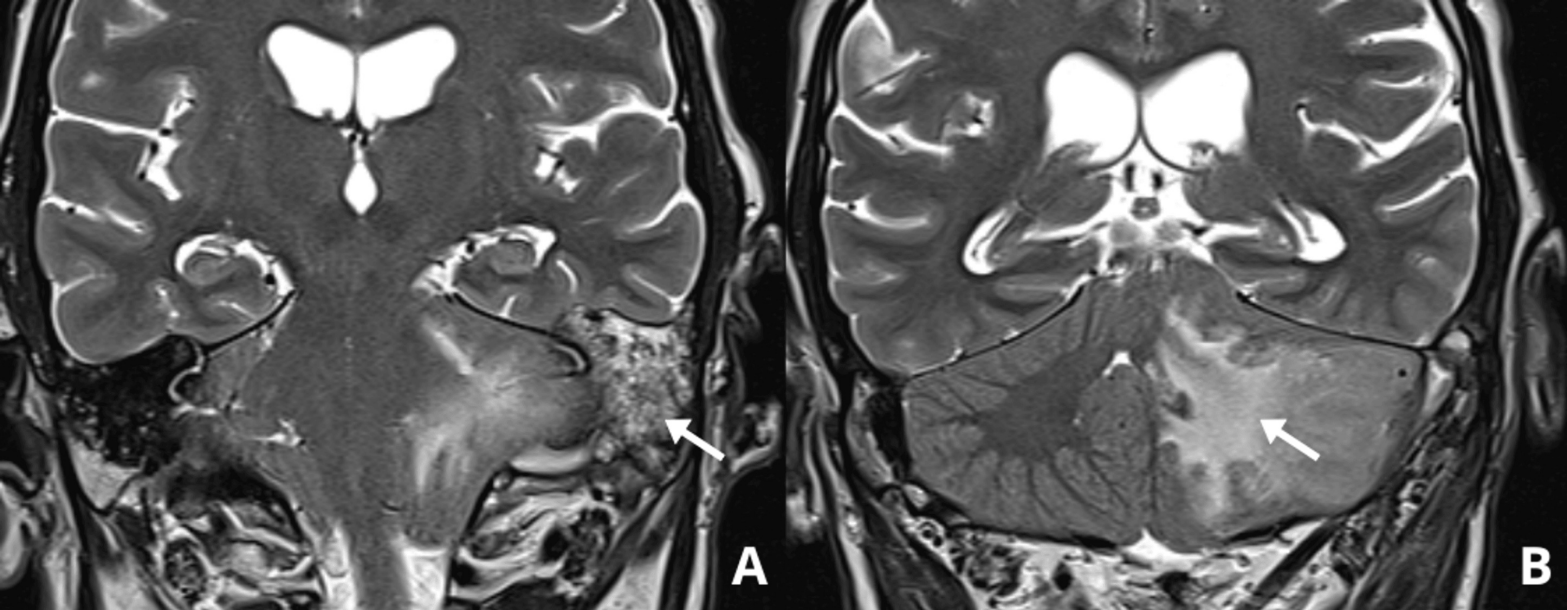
Brain MRI after six cycles of chemotherapy (coronal view) In the comparative study, progressive growth of a heterogeneous lesion (2.6 x 1.6 cm) occupying the left external auditory canal and filling the mastoid (arrow in panel A). A new expansive lesion in the left cerebellar hemisphere, measuring 3.7 cm (arrow in panel B), causing compression of the fourth ventricle.
